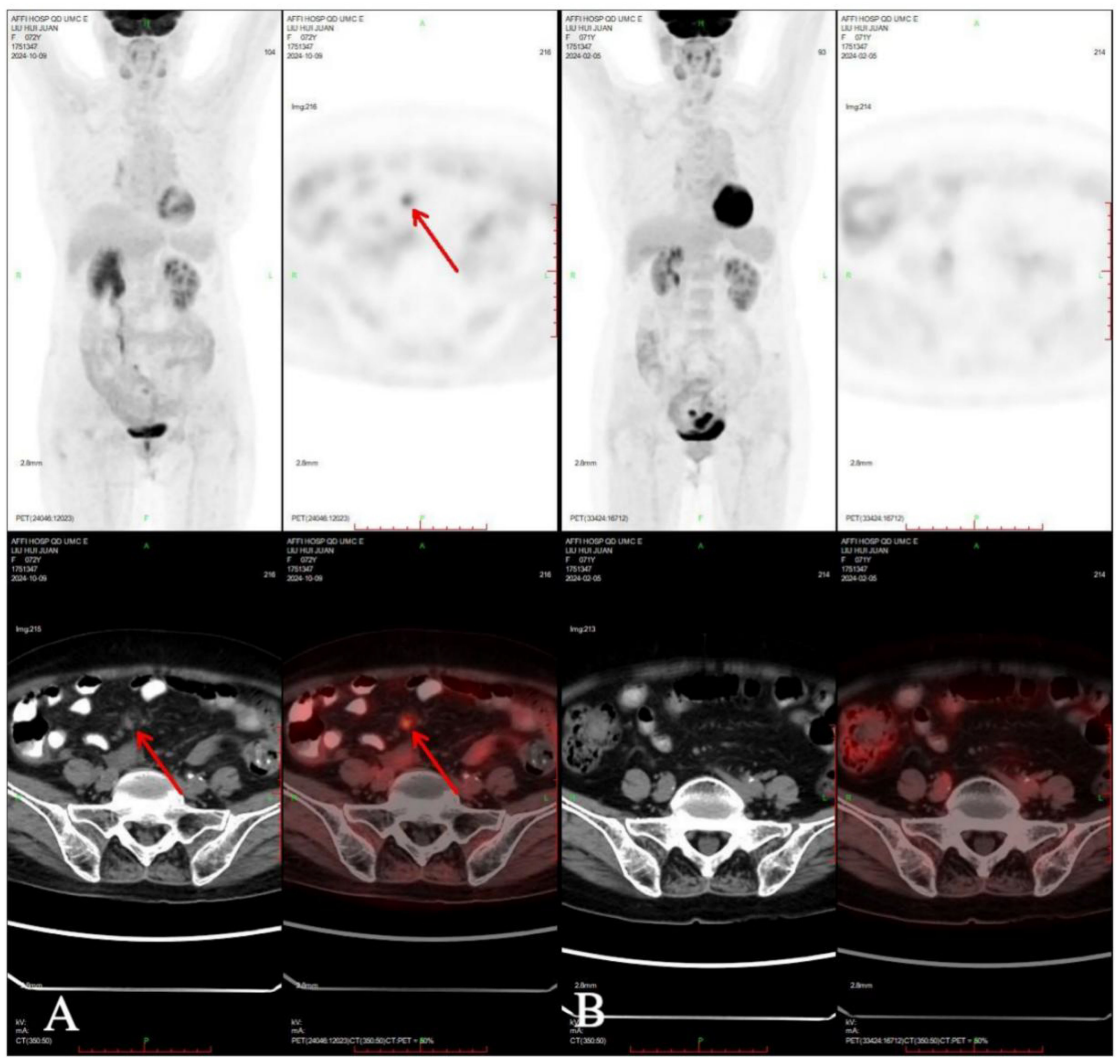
Compared PET-CT (A) in 2024-10-09 with PET-CT (B) in 2024-02-05, new lesions were found in the omentum.
